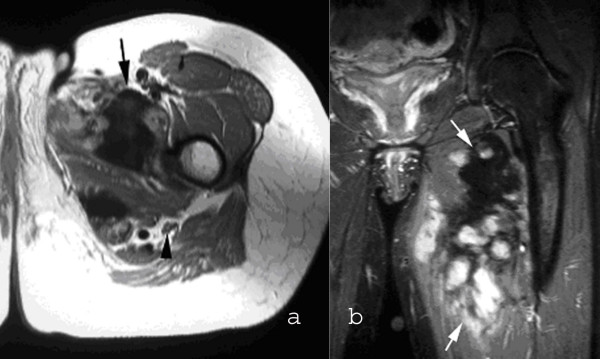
MRI of the left thigh. Axial T1W SE (a) and coronal STIR (b) images showing a poorly defined, lobular mass in the left adductor compartment (arrows) showing extensive areas of signal void due to fibrous tissue. Note the location of the sciatic nerve (arrowhead).
